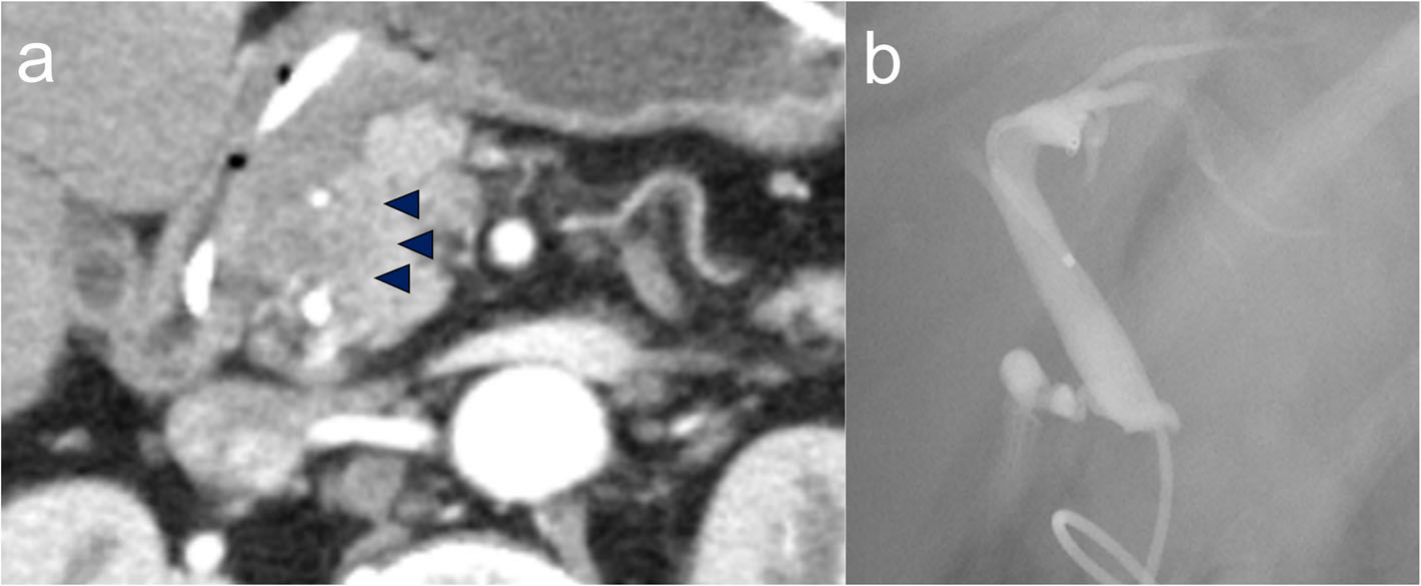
a Enhanced CT. Early phase of CT shows a low-density mass approximately 2 cm from the extrahepatic bile duct (black arrowheads). b Endoscopic retrograde cholangiography (ERC). ERC was performed and showed a long defect in the lower bile duct, and a tube stent was inserted
